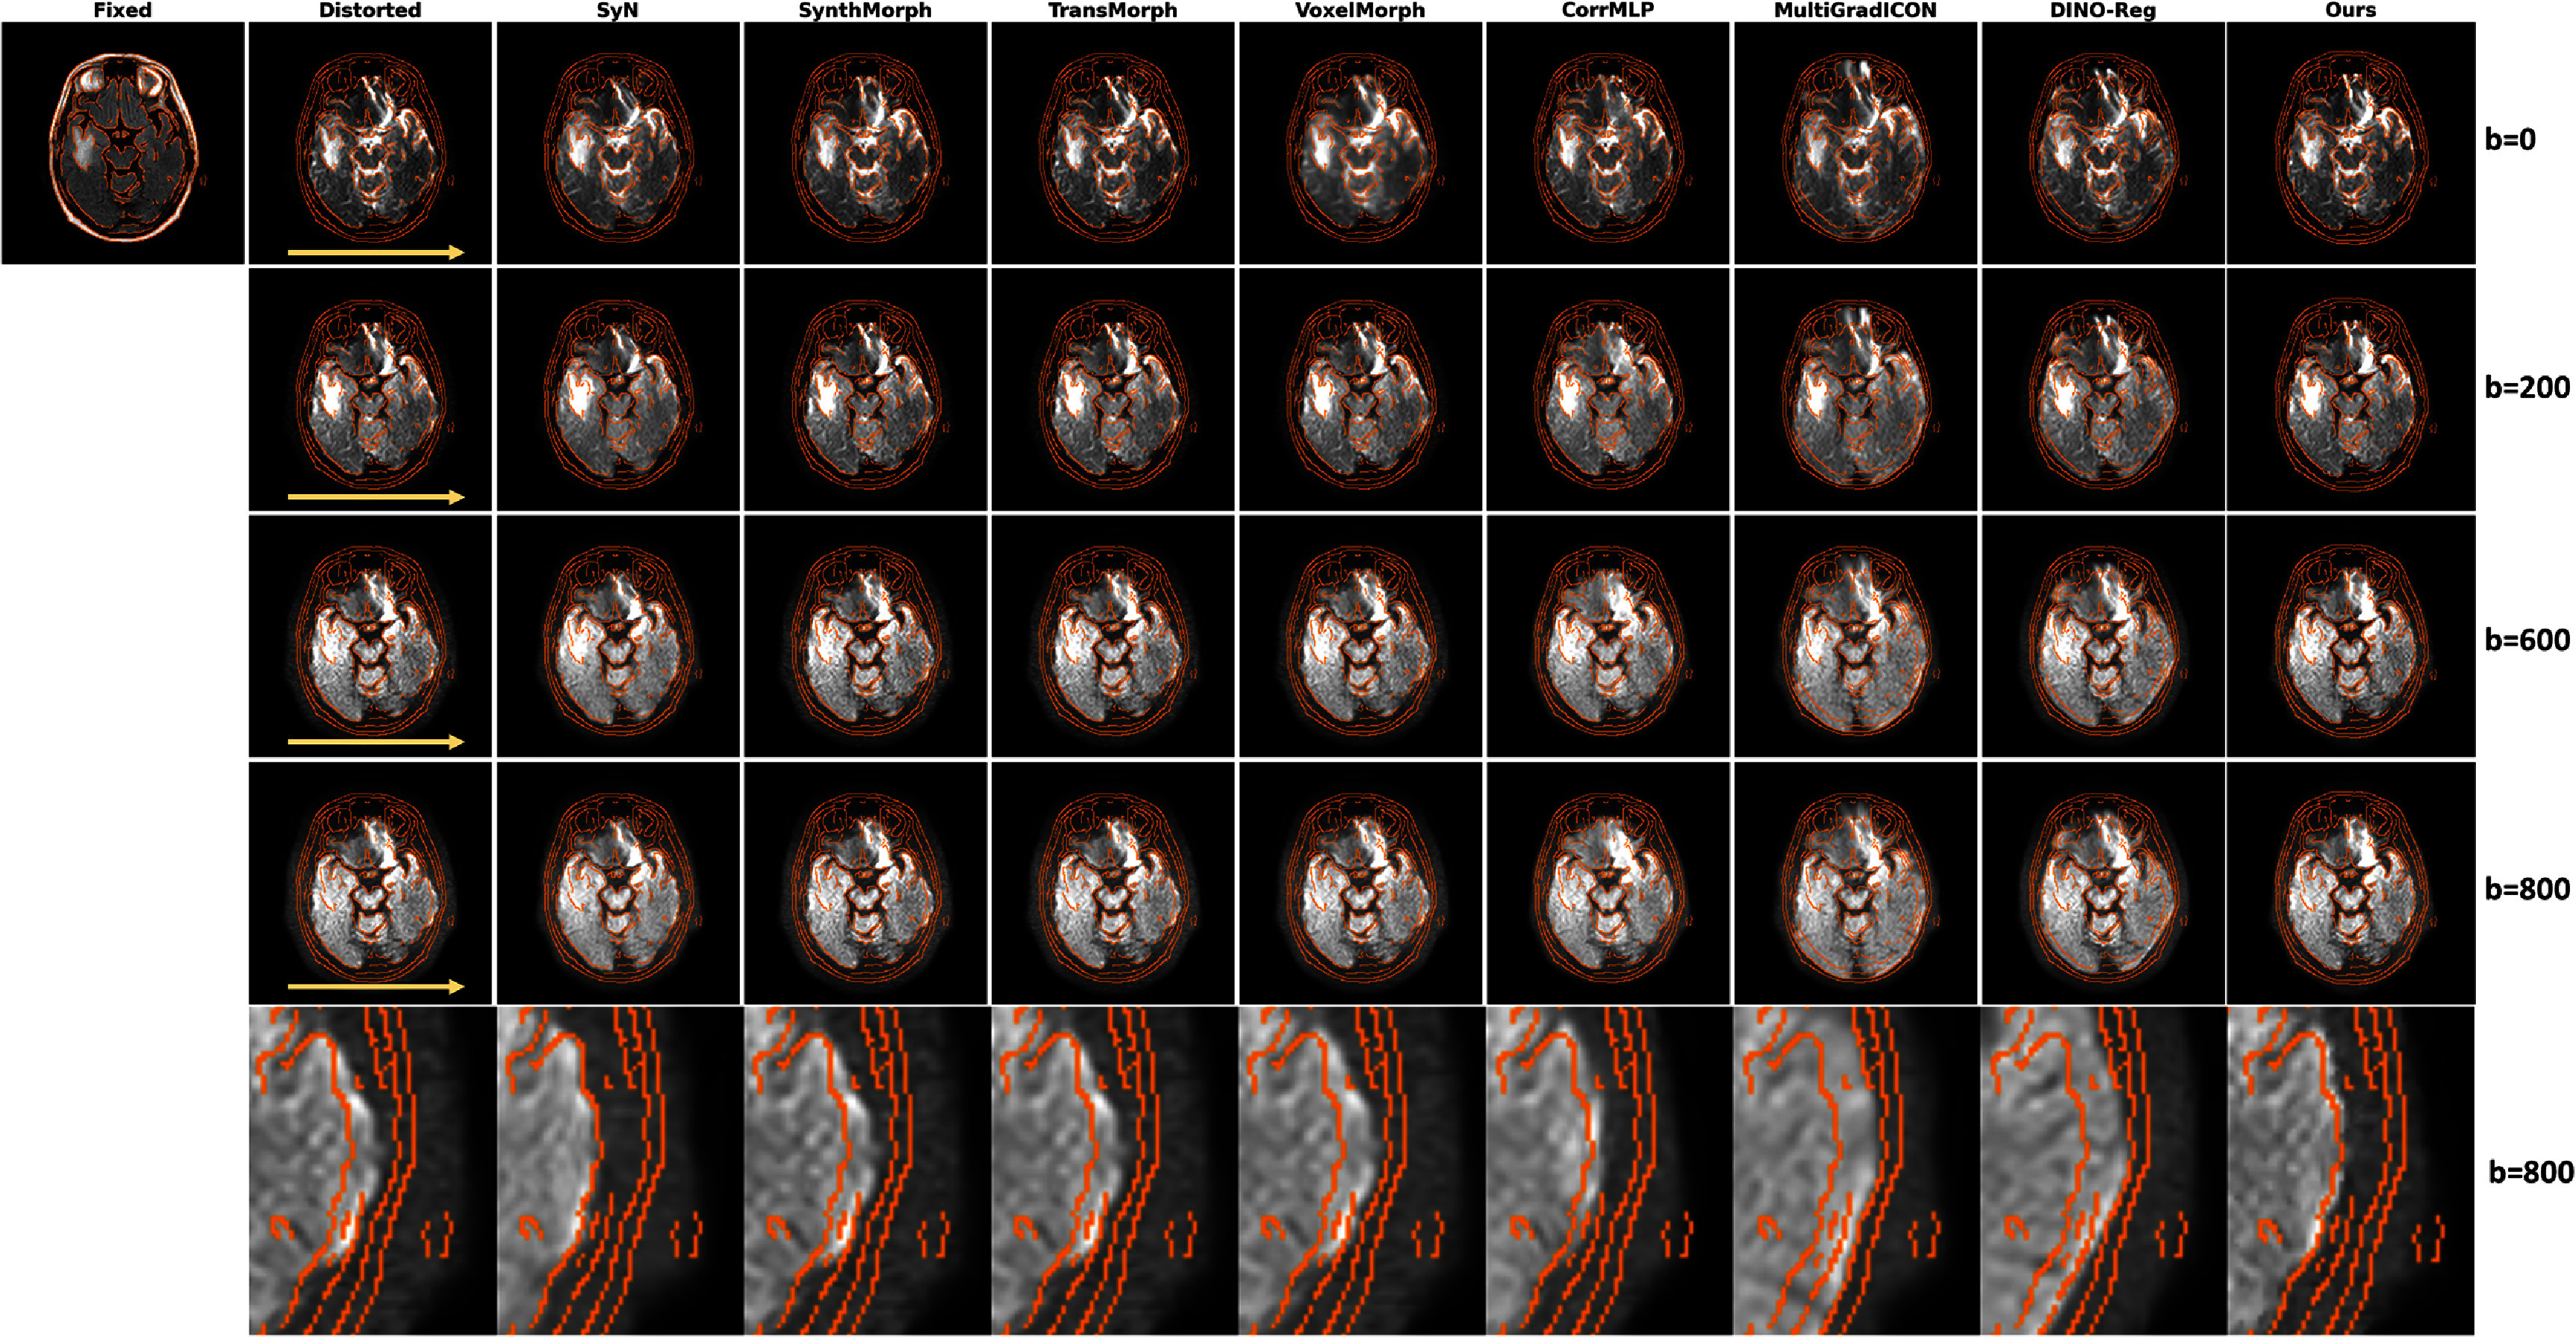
Multi-*b*-value registration results on brain DWI. Rows represent different *b*-values (*b* = 0, *b* = 200, *b* = 600, and *b* = 800) with deformation fields estimated from *b* = 0 images and applied across all diffusion weightings. Orange contours extracted using Canny edge detection on the FLAIR reference highlight anatomical boundaries. Yellow arrows indicate the phase encoding direction.
